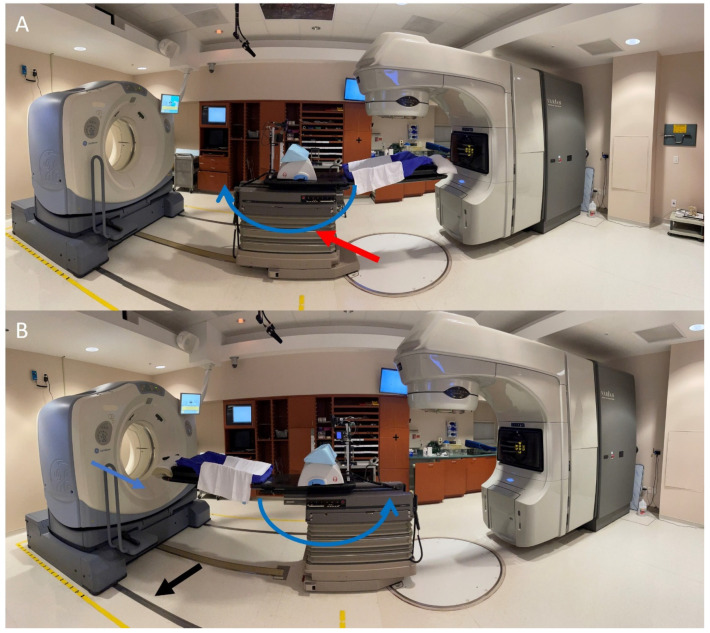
Photos of the CTOR–linac set up. First the patient is set up in the treatment position, with the couch positioned in the linac position (A) and BBs are placed in the laser positions. The base of the couch (red arrow) is then rotated 180° (curved blue arrows), according to the CT scanner position (B). The CT scanner moves along the rails on the floor (black arrow) in the direction of the straight blue arrow to take a scan. After image alignment, the patient is rotated back to the linac position and the lasers are aligned with the BBs. Final couch shifts are made from this position.
